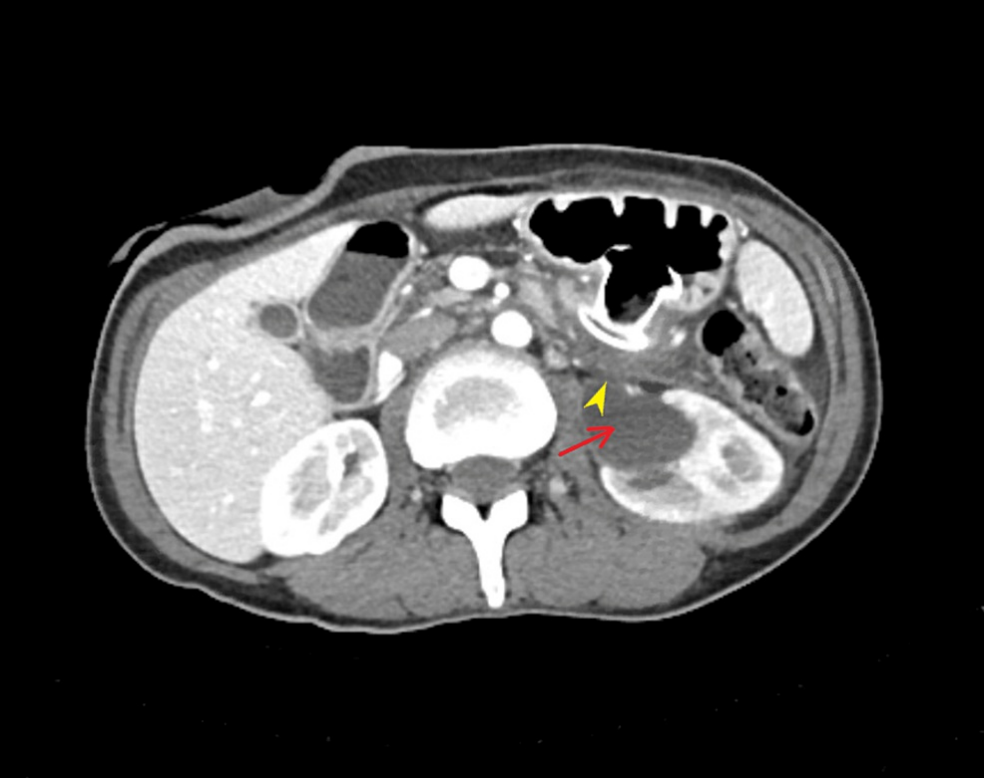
CT scan of the abdomen with IV contrast showing left-sided hydronephrosis (red arrow) and close apposition of the left ureter with jejunum (yellow arrowhead)
